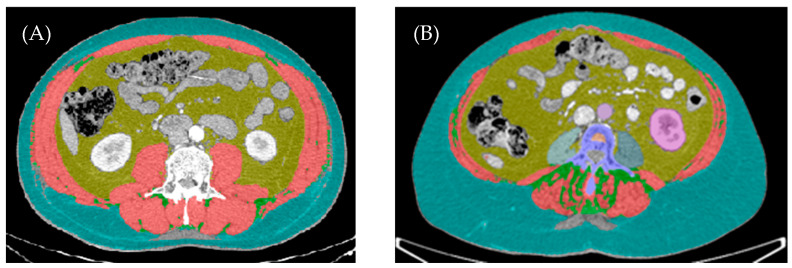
CT scan assessment at the L3 vertebral using DAFS software. Red—skeletal muscle area. Yellow—visceral adipose tissue area. Blue—subcutaneous adipose tissue area. Green—intramuscular adipose tissue area. Representation of 2 patients: (A) male, overweight (BMI 26.7 kg/m^2^) without sarcopenia/high muscle quantity (SMI 67.8 cm^2^/m^2^), (B) female, obese (BMI 34.8 kg/m^2^) with sarcopenia (SMI 36.8 cm^2^/m^2^).
